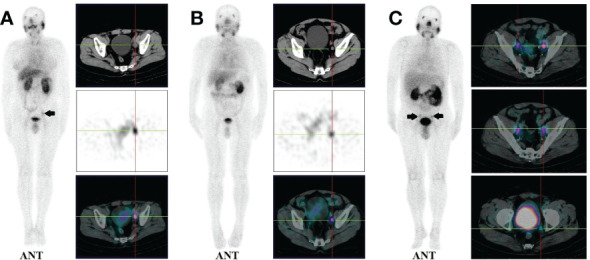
(A): Images of biochemically recurrent prostate cancer in a 72-year-old male with rising PSA levels after radical prostatectomy (PSA, 3.43 ng/ml; Gleason score, 4 + 4). Whole-body planar scintigraphy (pointed by black arrow) and transaxial SPECT/CT fusion images indicated a diameter of 13 mm lymph node metastases near the left iliac blood vessels, which shows pathological tracer uptake (SUVmax, 3.67). Final pathology confirmed prostate cancer metastasis. (B): Images of biochemically recurrent prostate cancer in a 61-year-old male with rising PSA levels after radical prostatectomy (PSA, 0.73 ng/ml; Gleason score, 4 + 5). Transaxial SPECT and fused SPECT/CT images show high 99mTc-HYNIC-PSMA uptake (SUVmax, 2.74) in subcentimeter (8 mm) lesions, as determined by corresponding CT. The patient underwent radiation therapy, and his PSA level decreased to 0.11 ng/ml. (C): Images of biochemically recurrent prostate cancer in a 70-year-old male with rising PSA levels after radical prostatectomy (PSA, 9.26 ng/ml; Gleason score, 4 + 4). Whole-body planar scintigraphy (pointed by black arrows) and transaxial SPECT/CT fusion images show multiple lymph node metastases (diameter between 7 and 12 mm) near the iliac blood vessels, which shows pathological tracer uptake (the highest SUVmax, 3.42). The patient received ADT, and his PSA level decreased to 0.53 ng/ml.
